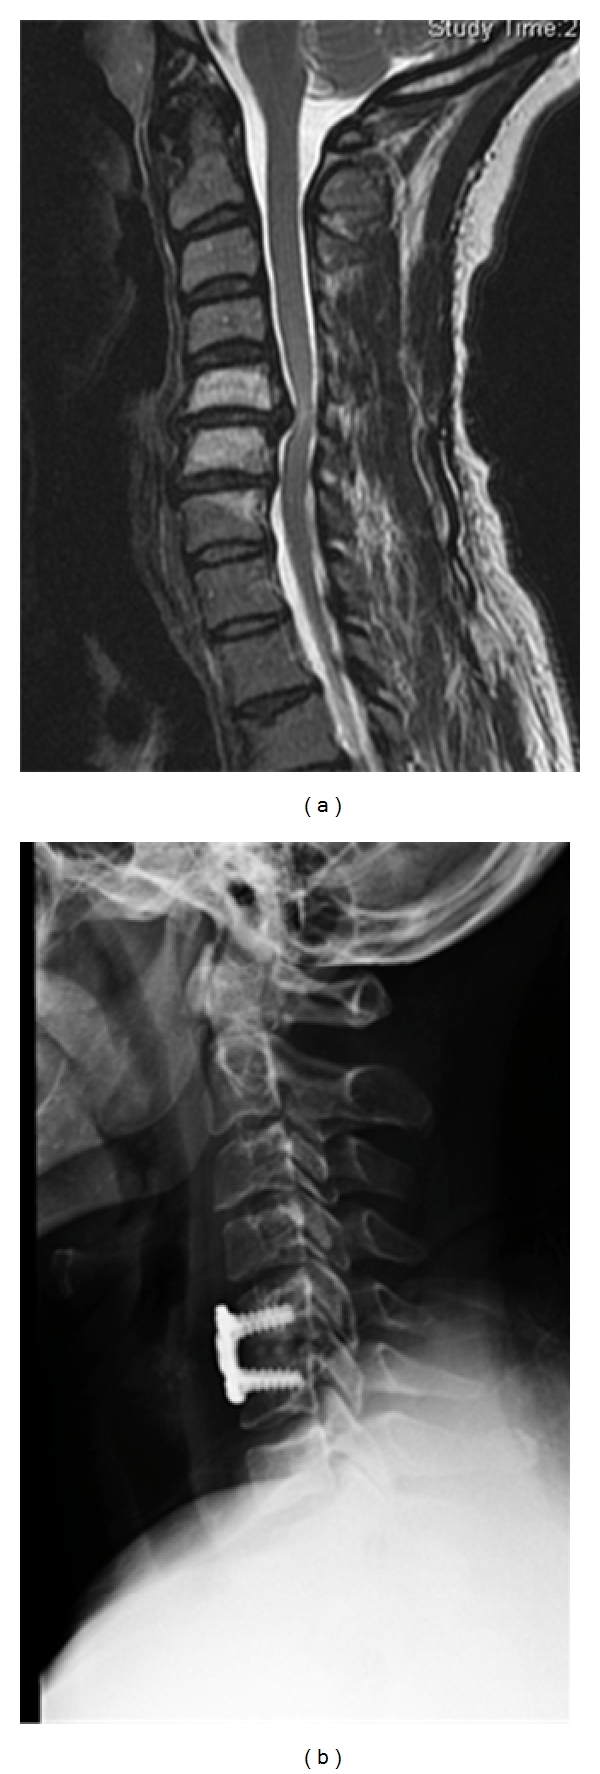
(a) Sagittal MRI demonstrating C5-6 extruded disc herniation. (b) Lateral radiograph of same patient after undergoing anterior cervical discectomy and fusion.
